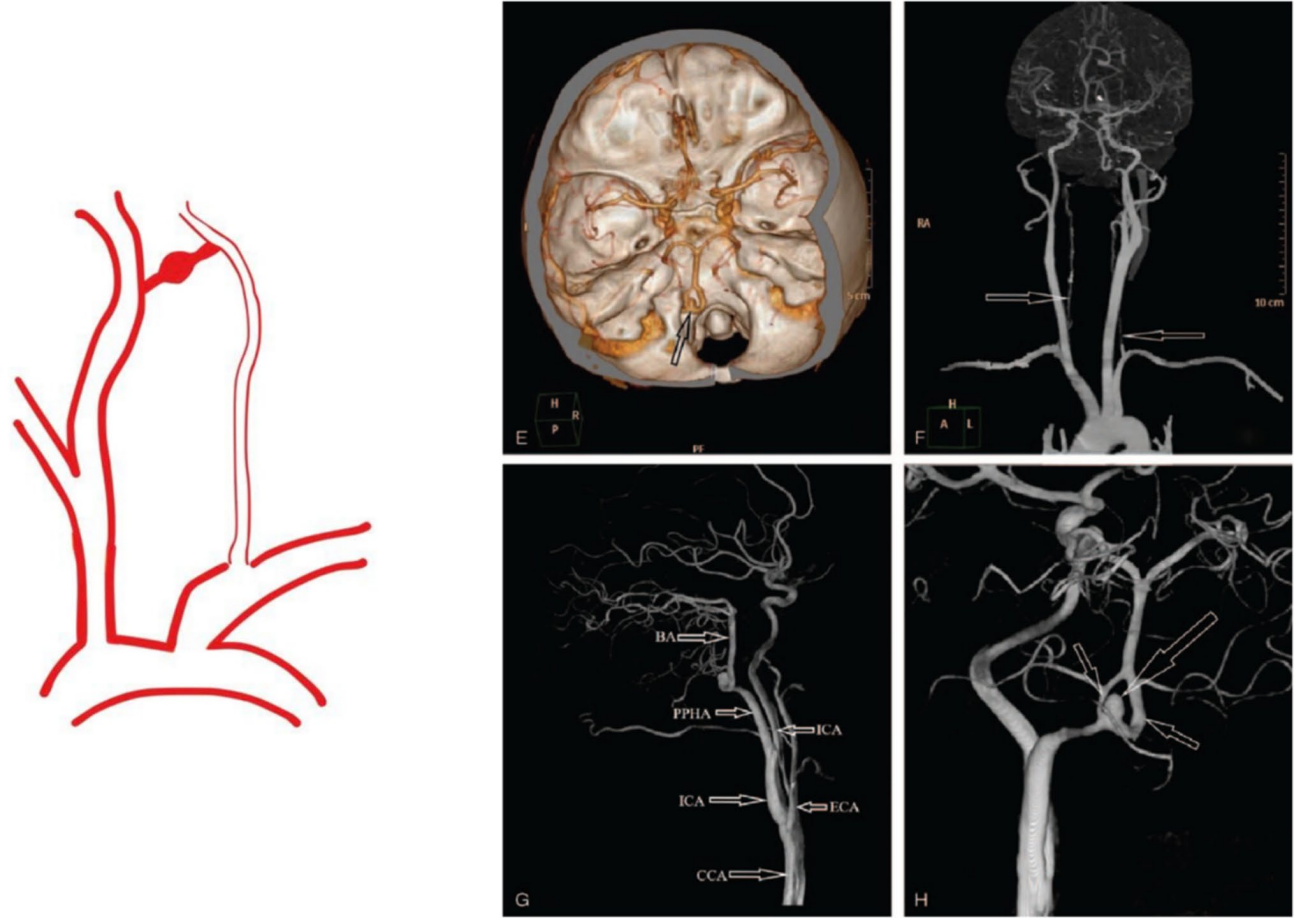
Category III PPHA: PPHA with aneurysm formation. Preoperative imaging (A–D) demonstrated intracranial hemorrhage and a persistent primitive hypoglossal artery (PPHA). Image A shows a CT scan revealing hemorrhage within the fourth ventricle, while image B demonstrates third and bilateral lateral ventricular hemorrhages. Image C identifies a PPHA arising from the internal carotid artery (ICA) at the level of the C1–C2 vertebrae, and image D shows its intracranial entry via the hypoglossal canal. Additional imaging (E–H) using CTA and DSA revealed a fenestrated vascular malformation with a cystic protrusion on the left side. Specifically, image E shows a fenestrated PPHA with a ruptured aneurysm and absent posterior communicating arteries; images F–H confirm hypoplastic vertebral arteries with no contribution to the basilar artery and a PPHA supplying the posterior circulation, including an associated aneurysm and vascular fenestration [17]. Reproduced from He S et al., Medicine (Baltimore). 2021;100(32):e26904. Used under the Creative Commons Attribution (CC BY 4.0) License
